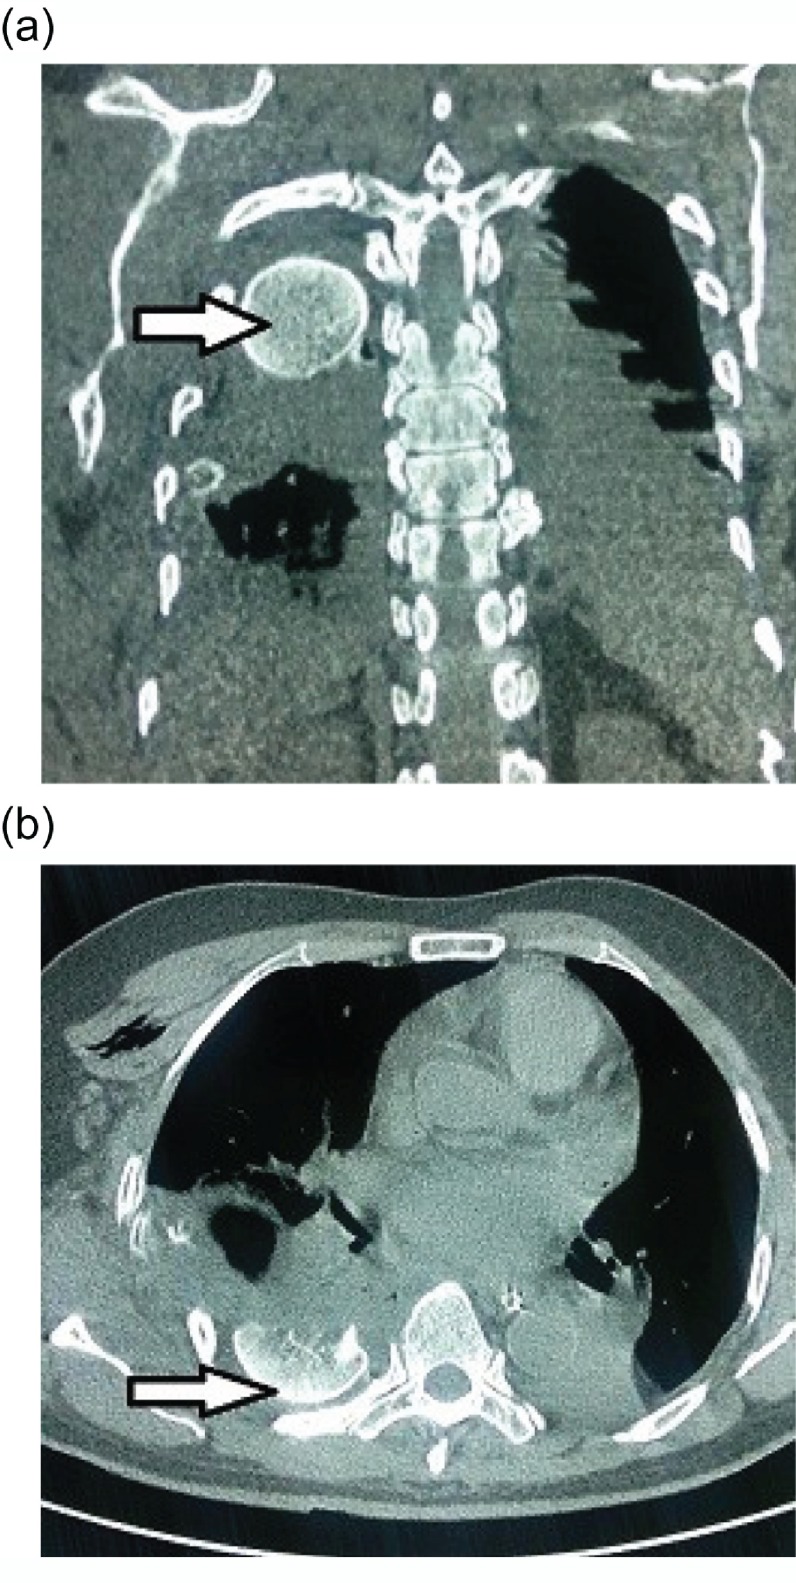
(a) Coronal plane CT showing intra-thoracic humeral head on right side. (b) Axial plane CT showing pleural effusion with right sided intrathoracic humeral head.
